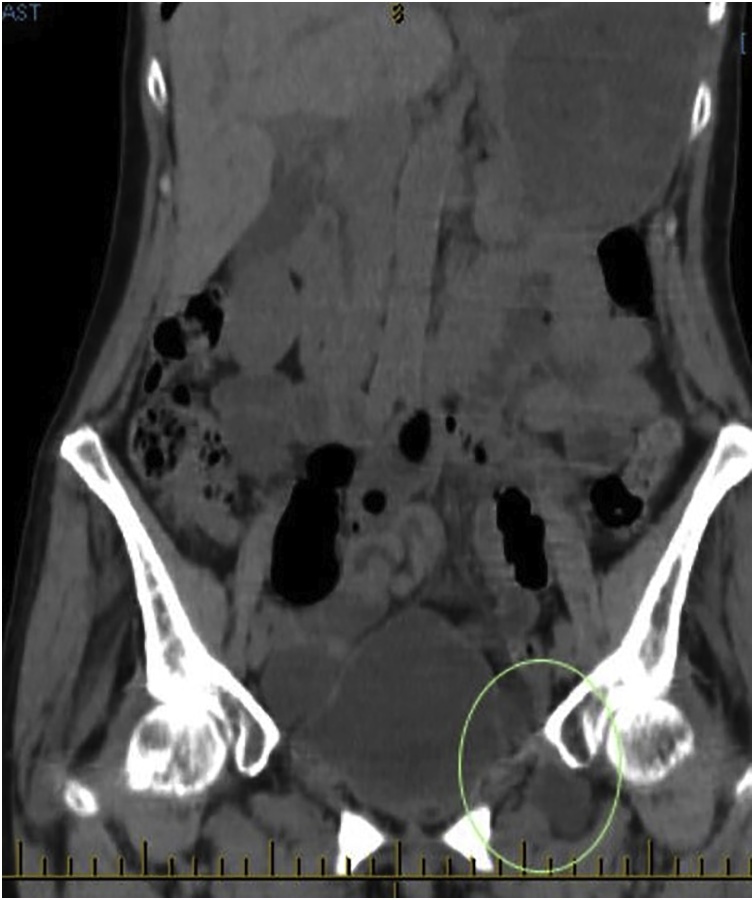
Coronal CT imaging demonstrating small bowel within the obturator canal.
